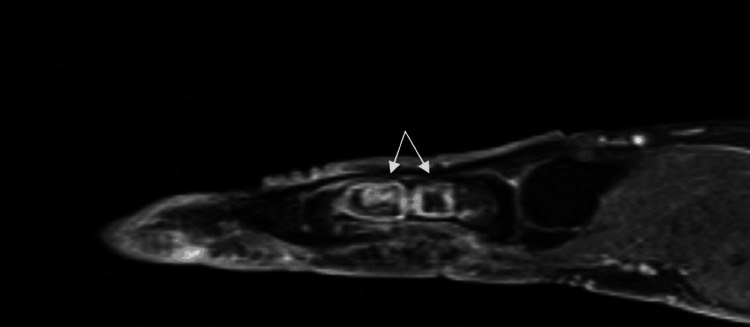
Preoperative MRI (T1 sagittal) indicating double intraosseous lesions in the proximal phalanx of the index finger (white arrows).
